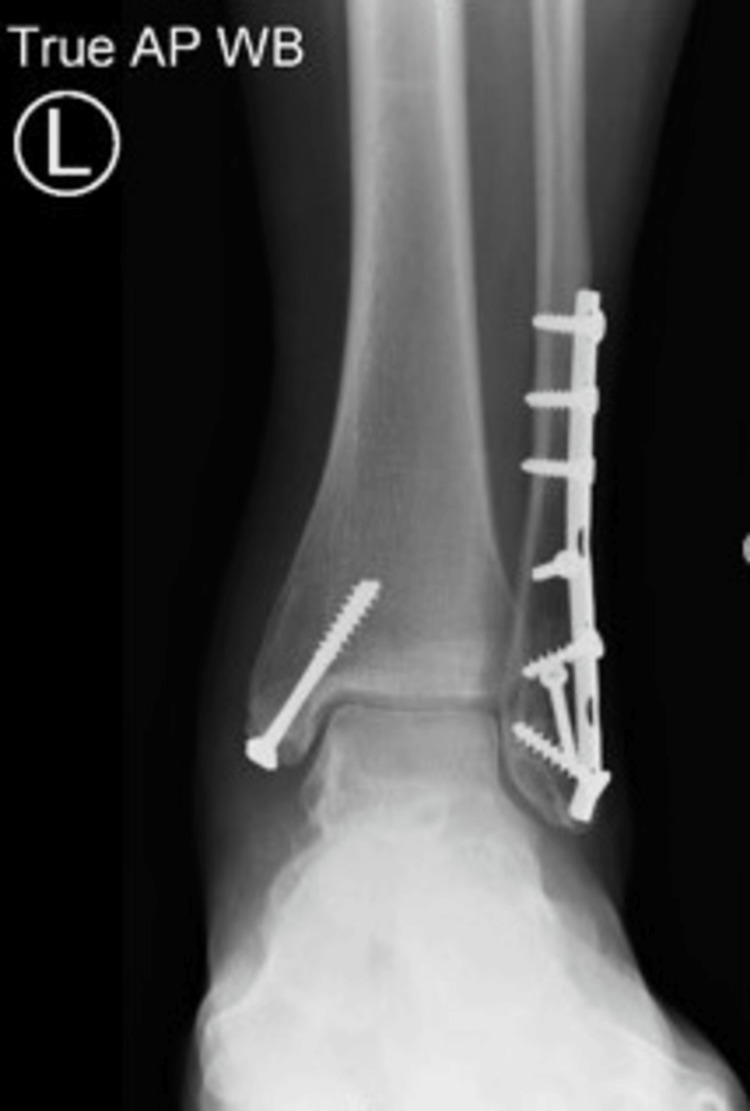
AP X-ray view of a bi-malleolar fracture treated with plate and screws showing good bony healing AP: anteroposterior
